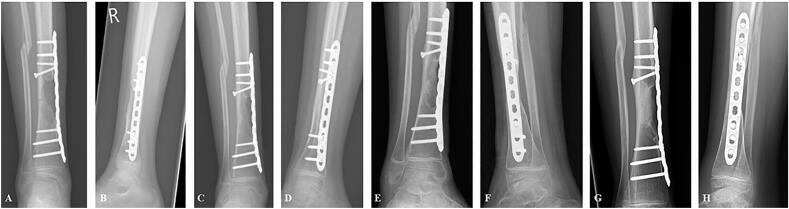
Postoperative X-rays showing increased callus formation and hypertrophy at the fracture site during follow-up; 1 month (A, B); 3 months (C, D); 6 months (E, F); 12 months (G, H).
